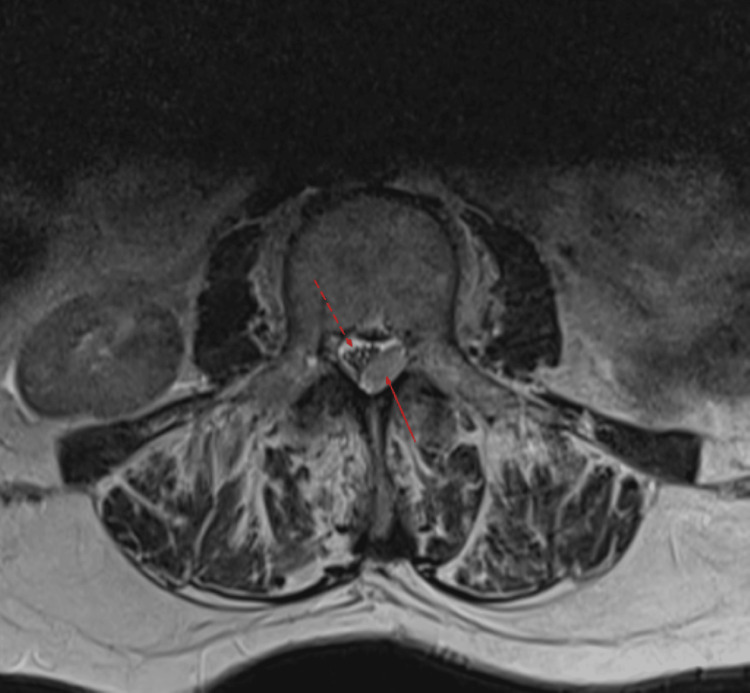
T2 axial MRI demonstrates mass within the spinal canal (solid arrow) displacing the cord anterolaterally (dashed arrow). MRI: magnetic resonance imaging
